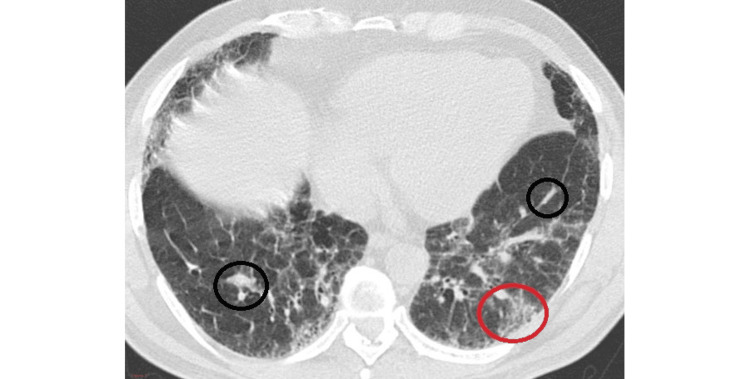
High-resolution computed tomography pattern inconsistent with UIP – demonstrating patchy ground glass (red circle) and peripheral reticular opacities (black circles) Source: Sverzellati [22]
